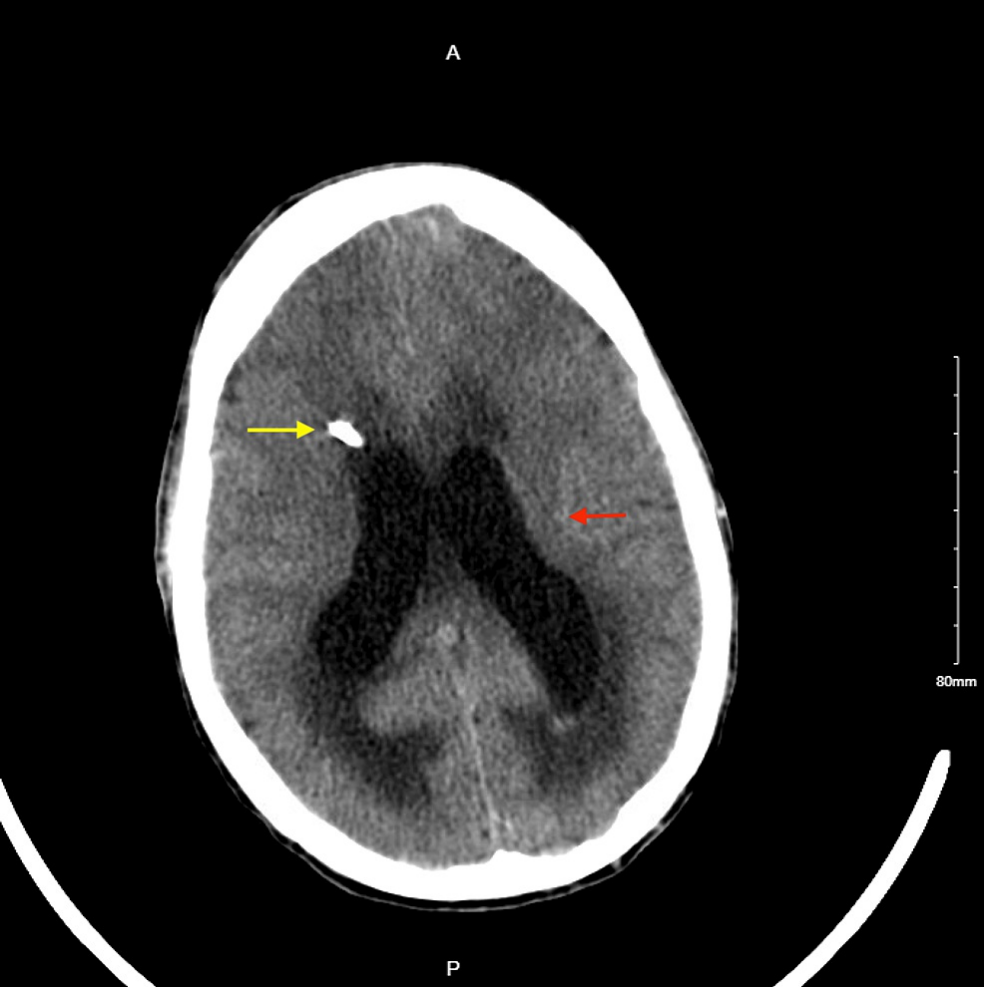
CT head post-external ventricular drain placement: ventricular drain seen entering from the right frontal calvarium into the third ventricle (yellow arrow), interval decreased dilation of the lateral ventricles, and decreased subependymal edema (red arrow).
